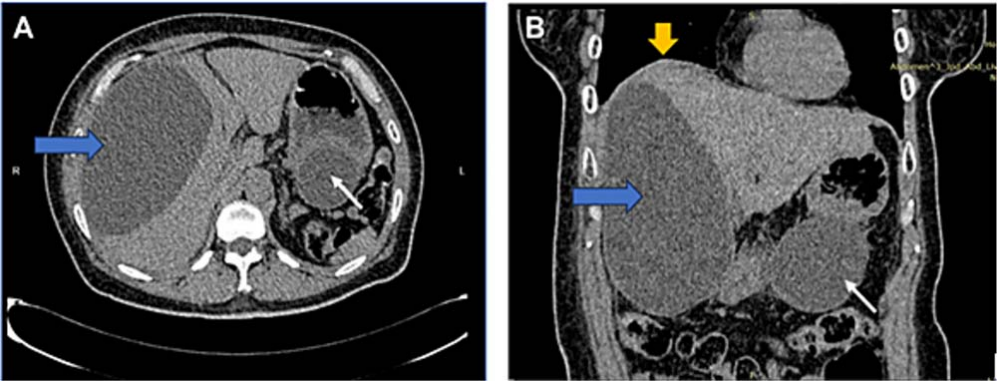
CT image: Axial (A) and coronal (B) view showing a well-defined, homogenous cystic lesion (white arrow) arising from the greater curvature of the stomach. Dimensions were ~ 62/79/147 mm with evidence of external compression to the cardia, fundus, and greater curvature. Another large cystic mass (horizontal arrow) originated from the right liver lobe, measuring 91/146/172 mm. Mass effect was noted with superior displacement of the right hemidiaphragm (vertical arrow). No suspicious or aggressive features of these cystic lesions.
